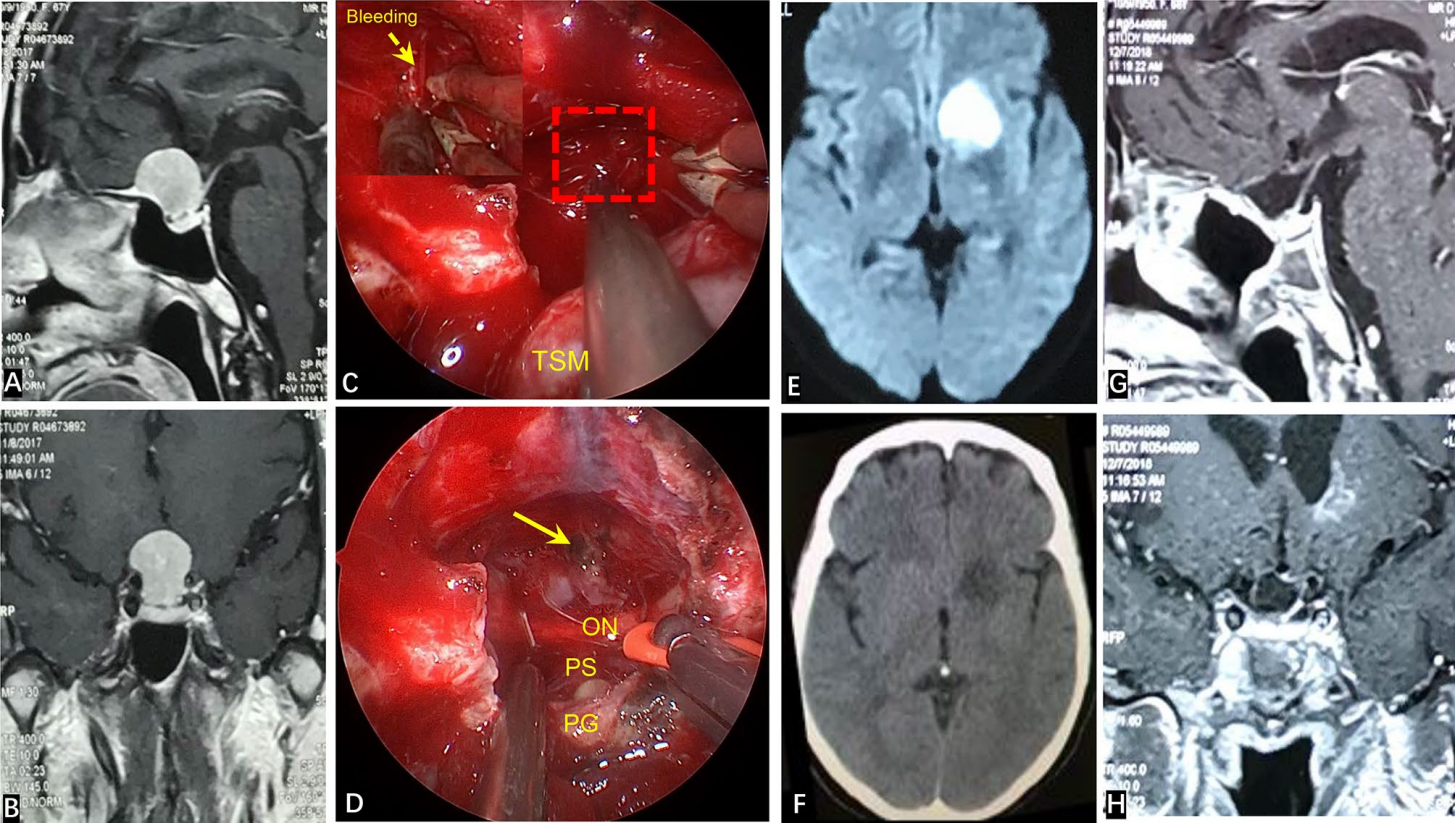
(A,B) Preoperatively, contrast-enhanced MRI of the sellar area showed the dimeter and shape of the tumor. (C) The texture of this tumor was tough, and bleeding occurred in the A2 branch of the ACA intraoperatively (red dotted line box is the bleeding area, and the upper left corner is an enlarged image of the bleeding). (D) GTR of the tumor after completion of electrocoagulation and hemostasis of the A2 branch of the ACA (yellow arrow). (E) Walking instability occurred at POD5. Diffusion-weighted imaging shows recent infarction of the left caudate nucleus head. (F) CT reexamination at POD10 reveals muscle strength returned to normal and the patient could move freely. (G,H) One year after surgery, contrast-enhanced MRI of the sellar region showed that the tumor had been completely resected, and that there was no sign of residual tumor or recurrence.
